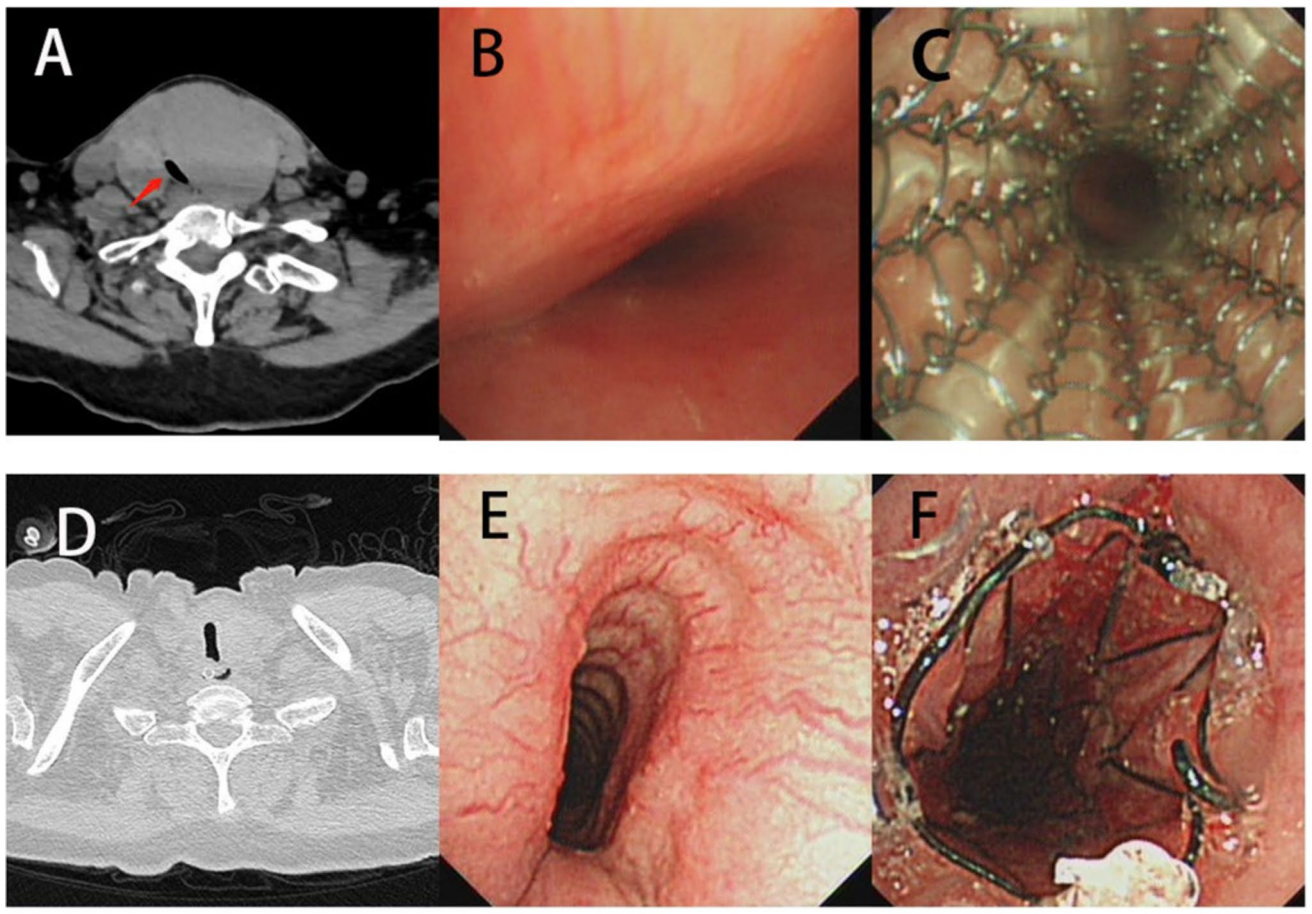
Benign airway stenosis caused by external compression. (A-C) CT and bronchoscopy characteristics of a 58-year-old woman, airway narrow was relieved after the plantation of a metallic stent. (D-F) CT and bronchoscopy characteristics of a 64-year-old woman, airway narrow was relieved after the plantation of a metallic stent.
